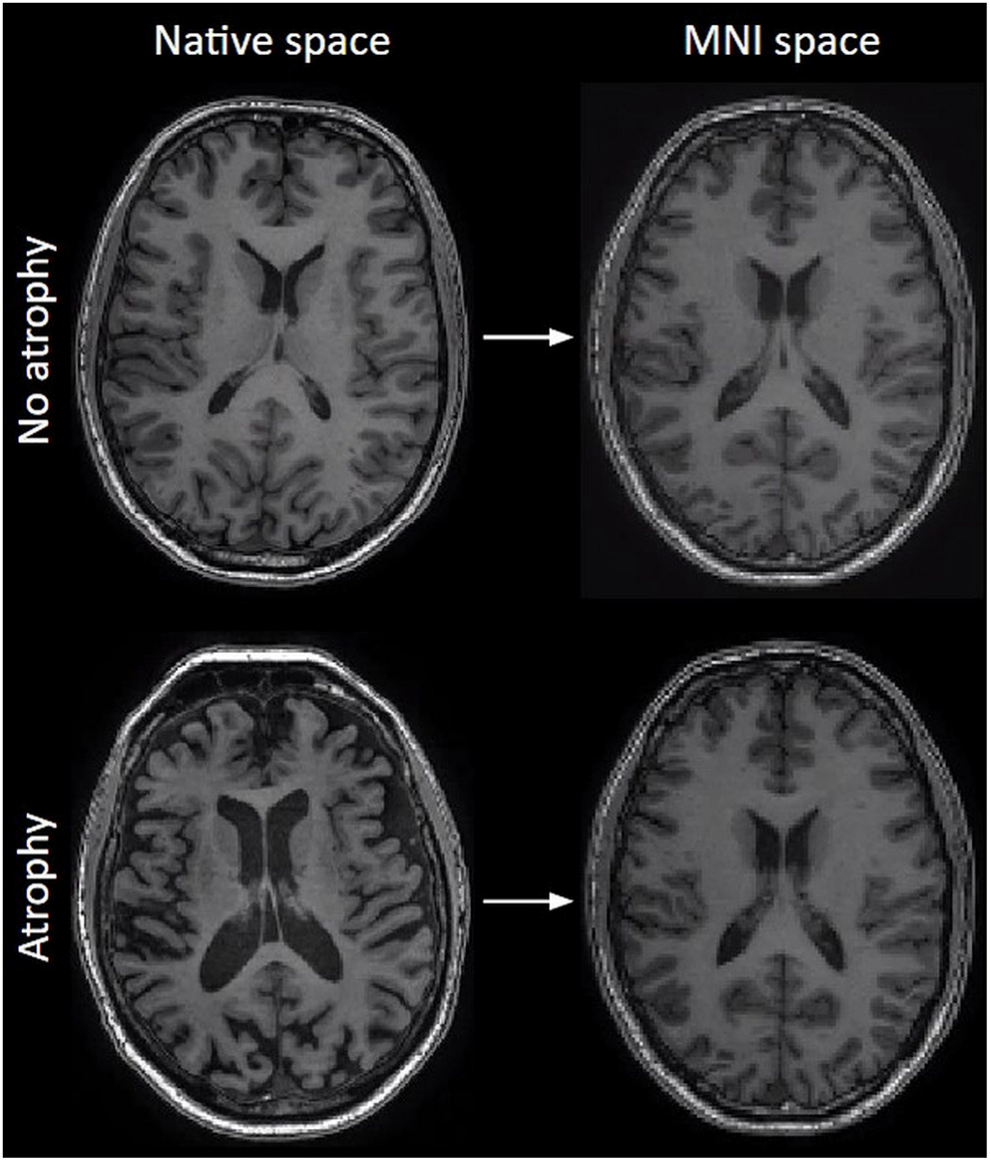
The effect of normalization on T1-weighted images. Left column: original images before normalization (native space), right column: after normalization to MNI space. Note how size differences and atrophy are largely compensated.
